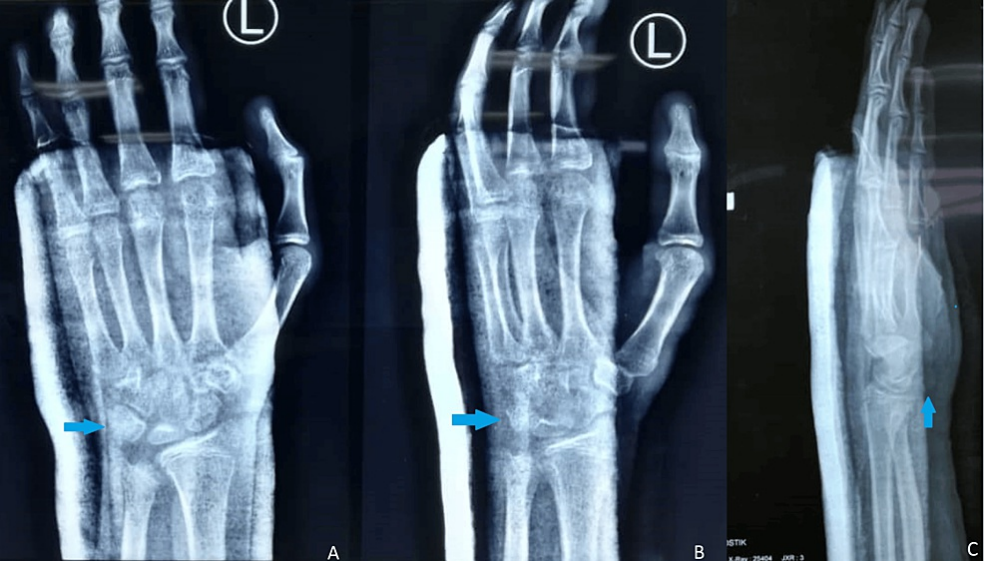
Postoperative plain radiographs showing the relocation of the pisiform bone (blue arrow) in (A) AP view, (B) oblique view, and (C) true lateral view. AP, anteroposterior
